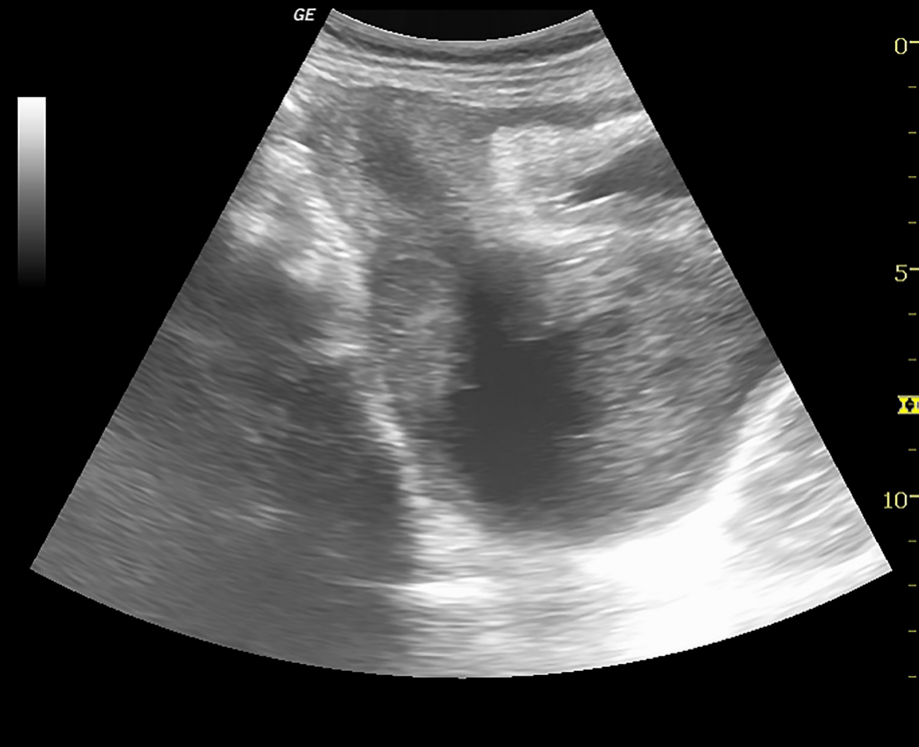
Longitudinal ultrasound image depicting a cystic lesion
